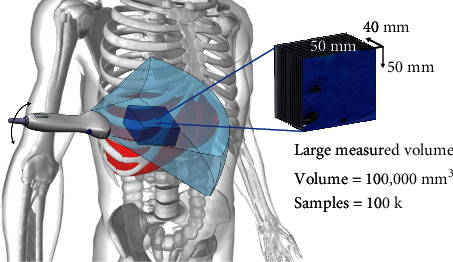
Velacur ultrasound probe is placed between the ribs to view the right lobe of the liver using an intercostal approach. A rendering of the general positioning of the probe, liver, and measured volume. The blue cone shows how much of the liver can be seen in the ultrasound image. The cube within the liver shows the large region that is used to measure the average elasticity and attenuation.
